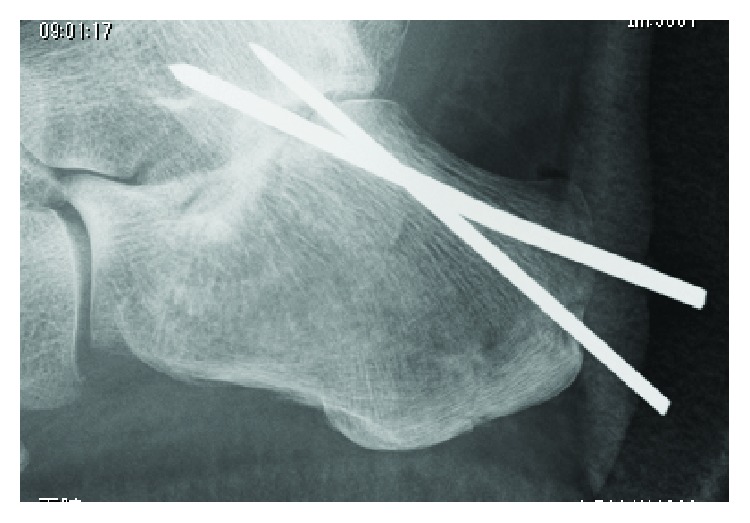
Lateral radiograph of the left foot following the first surgery. X-ray shows that the left calcaneus was almost completely reduced with two Steinmann pins.
